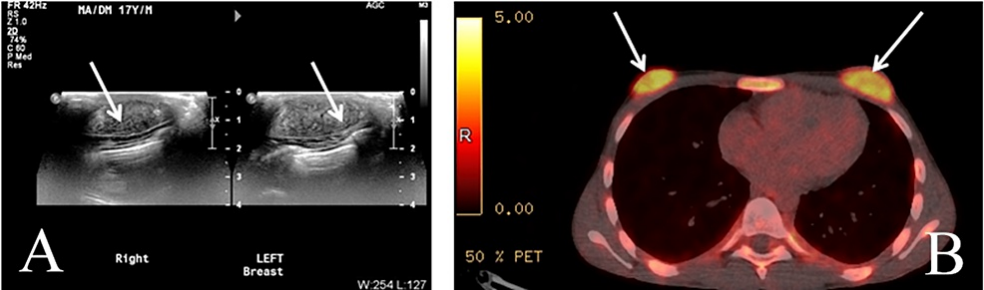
A 17-year-old male in remission from lymphoblastic leukemia when he developed bilateral breast swelling. The clinical suspicion was gynecomastia. Bilateral breast ultrasound revealed bilateral symmetrical well-defined heterogeneous retroareolar concentric masses also revealed in PET CT as bilateral symmetric retroareolar masses. Due to the clinical setting of leukemia, leukemic infiltrates were suspected and coded as BI-RADS 4C. A bilateral biopsy revealed lymphoblastic leukemia. (A) Bilateral retroareolar ultrasound. (B) PET CT.
